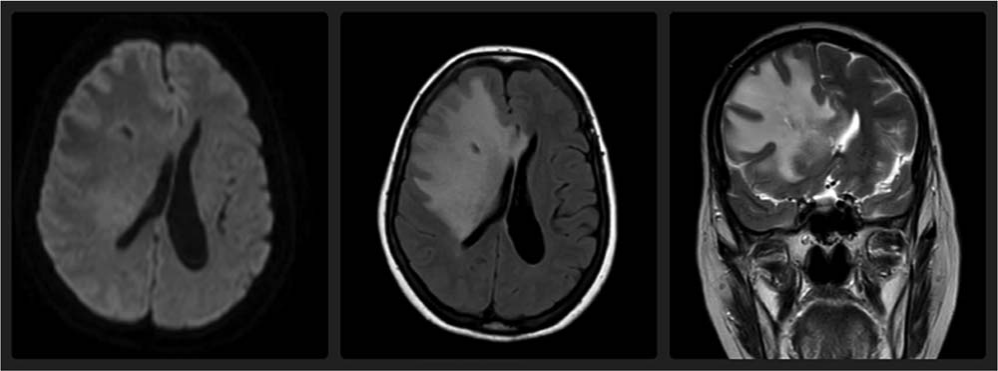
Axial FLAIR, axial DWI, and coronal T2-weighted MRI demonstrate a hyperintense signal in the right frontal lobe white matter on FLAIR and T2 sequences, without diffusion restriction, consistent with vasogenic edema and early leukoencephalopathy.
